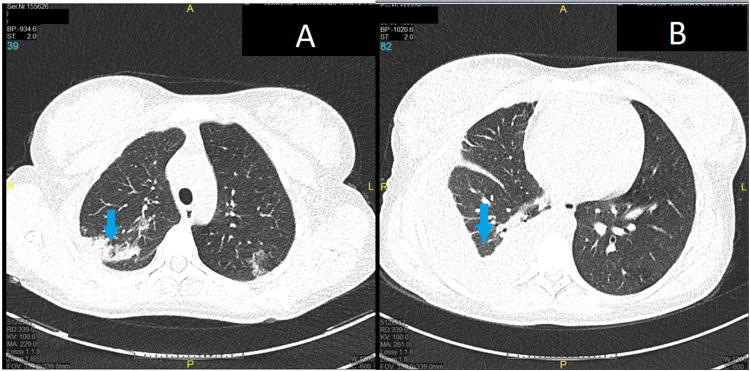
Low-dose CT of the lungs showing bilateral pulmonary consolidation (A) CT slide showing bilateral pulmonary consolidation predominantly on the right side (blue arrow). (B) CT slide showing pleural effusion (blue arrow).
